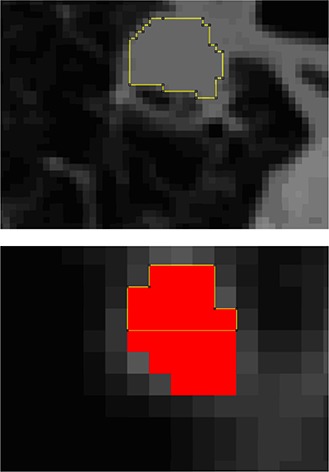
Segmentation of lesion #51: on CT image (a), and on the FDG PET image (in red) (b) where the segment outlined in yellow represents the area segmented on CT image (a).
